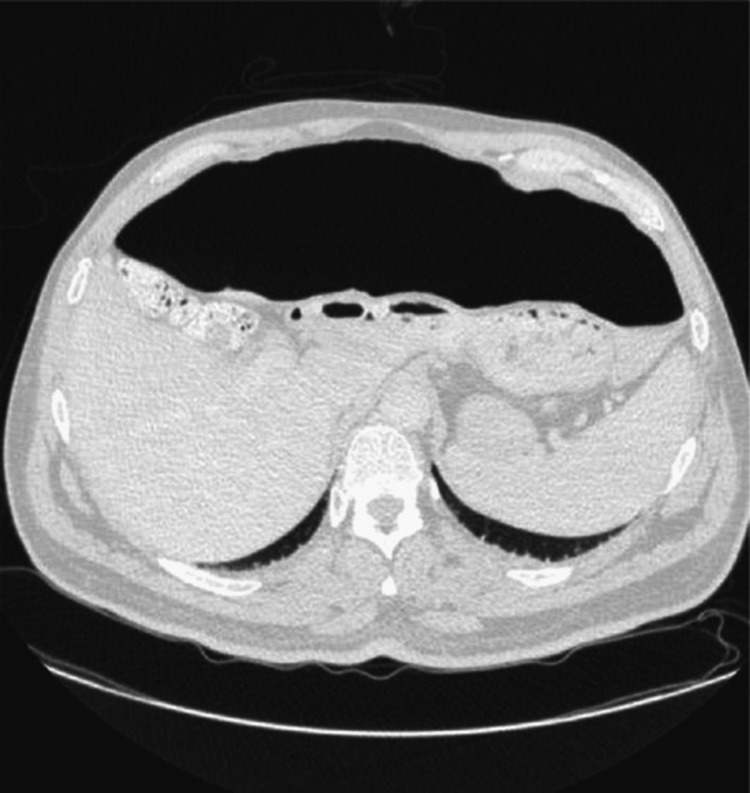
CT of the abdomen with contrast showing bowel distension and no other pathology
